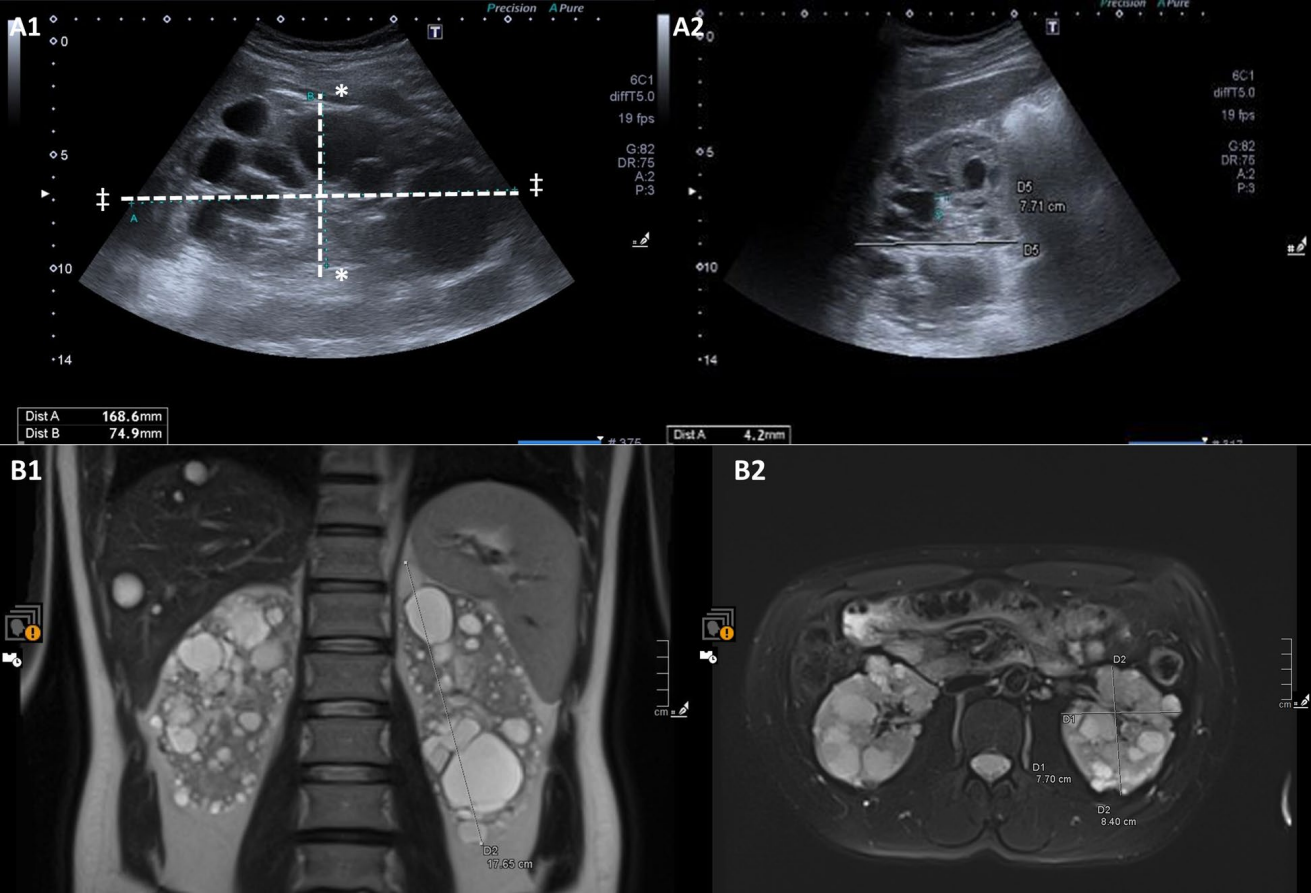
Kidney volume measurement of a 39-year-old male patient with autosomal-dominant polycystic kidney disease (ADPKD). A Kidney Volume Measurement Using Ultrasound. 1. Right Kidney Cranio-Caudal and Anterior–Posterior Diameters (Ellipsoid Formula). Cranio-Caudal Distance (marked * to *): 74.9.mm. Anterior–Posterior Distance (marked ‡ to ‡): 168.6 mm. 2. Right Kidney Antero-Posterior and Transverse Diameters (Ellipsoid Formula). Transverse Distance (D5): 77.1 mm. B Kidney Volume Measurement Using Magnetic Resonance Imaging (MRI). 1. MRI T2 Coronal Cranio-Caudal Diameters (Ellipsoid Formula). Cranio-Caudal Distance D1: 176.5 mm. 2. MRI T2 Axial Anterior–Posterior and Transverse diameter for ellipsoid formula. Anterior–Posterior Distance D2: 84.0 mm. Transverse Distance D1: 77.0 mm
